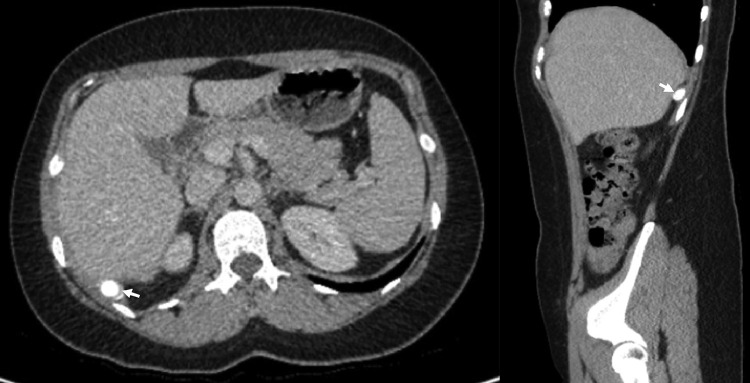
Axial (left) and sagittal (right) interval CT images obtained 4 years previously showing a 10 mm retrohepatic calcified body (white arrow).
